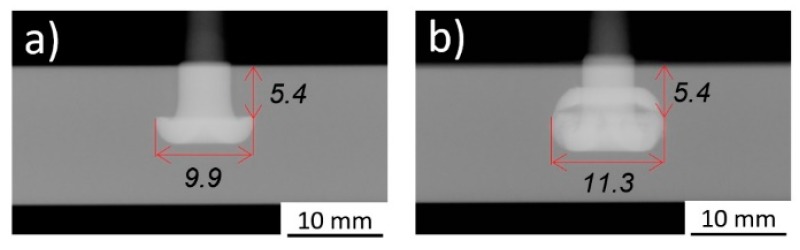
X-ray tomography of: (a) Condition 15 (RS = 18,000 rpm; FT = 2 s; FoT = 2 s; FF = 3000 N; FoF = 3900 N); and (b) Condition 12 (RS = 20,000 rpm; FT = 2 s; FoT = 1 s; FF = 3000 N; FoF = 3900 N).
